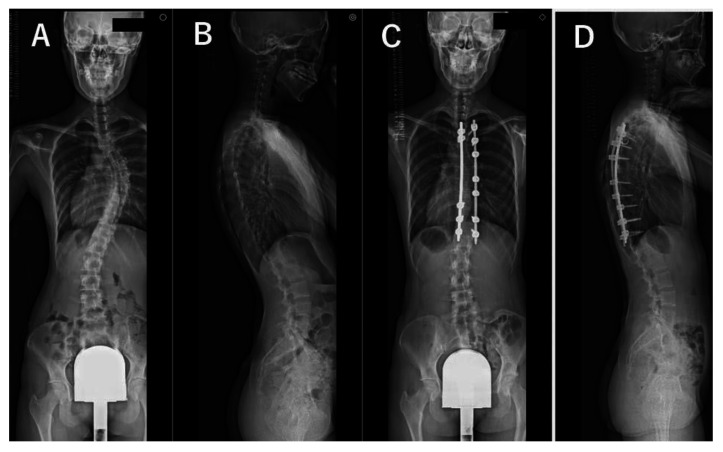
Pre- (A,B) and post- (C,D) operative radiographs of representative case with 20-year-old female patient. Cases of preoperative cervical hyperkyphosis changed to postoperative cervical lordosis. Cervical lordosis (−15°, 3°), thoracic kyphosis (20°, 28°), lumbar lordosis (59°, 52°), T1 slope (9°, 15°), major thoracic curve (51°, 17°). (Preoperative, postoperative).
